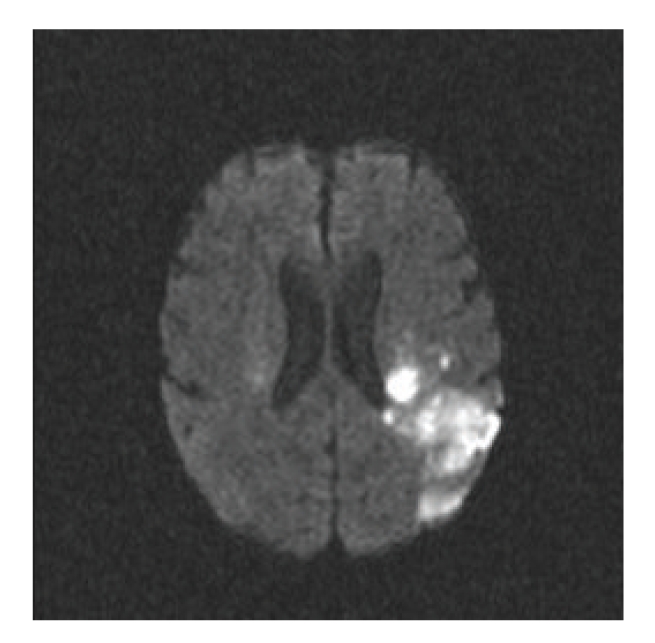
Diffusion weighted MRI after thrombolysis showing left parietal hyperintensities. Areas of focal intensities were also identified in other location including the right cerebral hemisphere, consistent with embolic source as a likely cause of these bilateral and sporadic hyperintense lesions.
